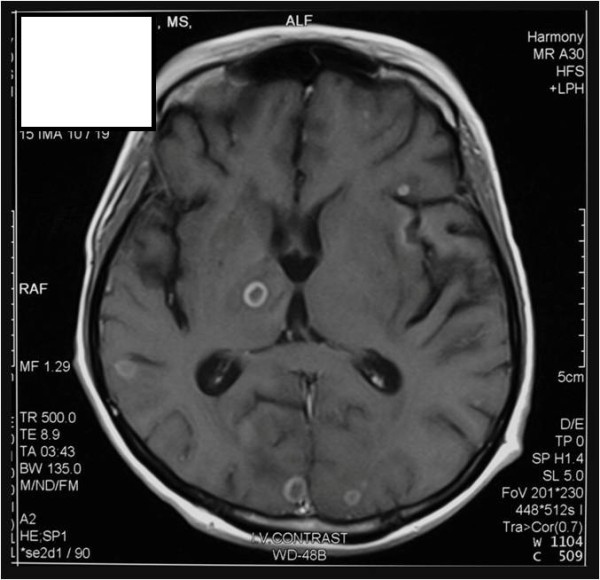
Magnetic resonance image after three months of anti-tuberculosis therapy. The image, which corresponds to that of Figure 1, shows a marked reduction in the number of lesions.
